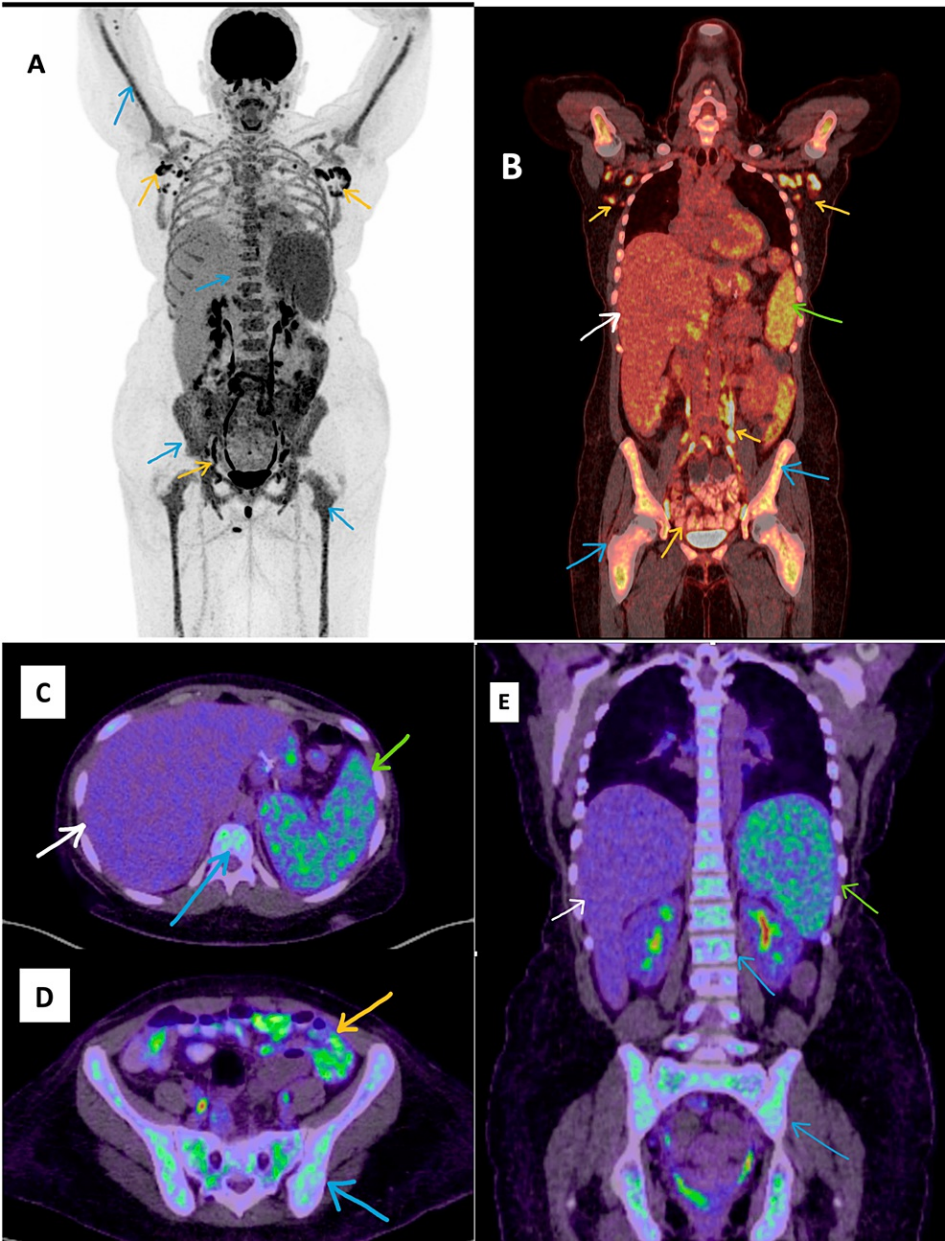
FDG-PET/CT scan with MIP image (A), fused coronal images (B,E), and fused axial images (C,D) Multiple hypermetabolic lymph nodes in the bilateral axillary, inguinal, iliac, para-aortic, aortocaval, and retroperitoneal regions (yellow arrows in A, B, and D). Marked hepatosplenomegaly with intense uptake in the spleen in B, C, and E (spleen shown as green arrows and liver shown as white arrows). Intense FDG uptake in the bone marrow, vertebra, and bilateral femurs and humerus in all images (blue arrows). FDG-PET/CT: fluorodeoxyglucose-positron emission tomography/computed tomography, MIP: maximum intensity projection
